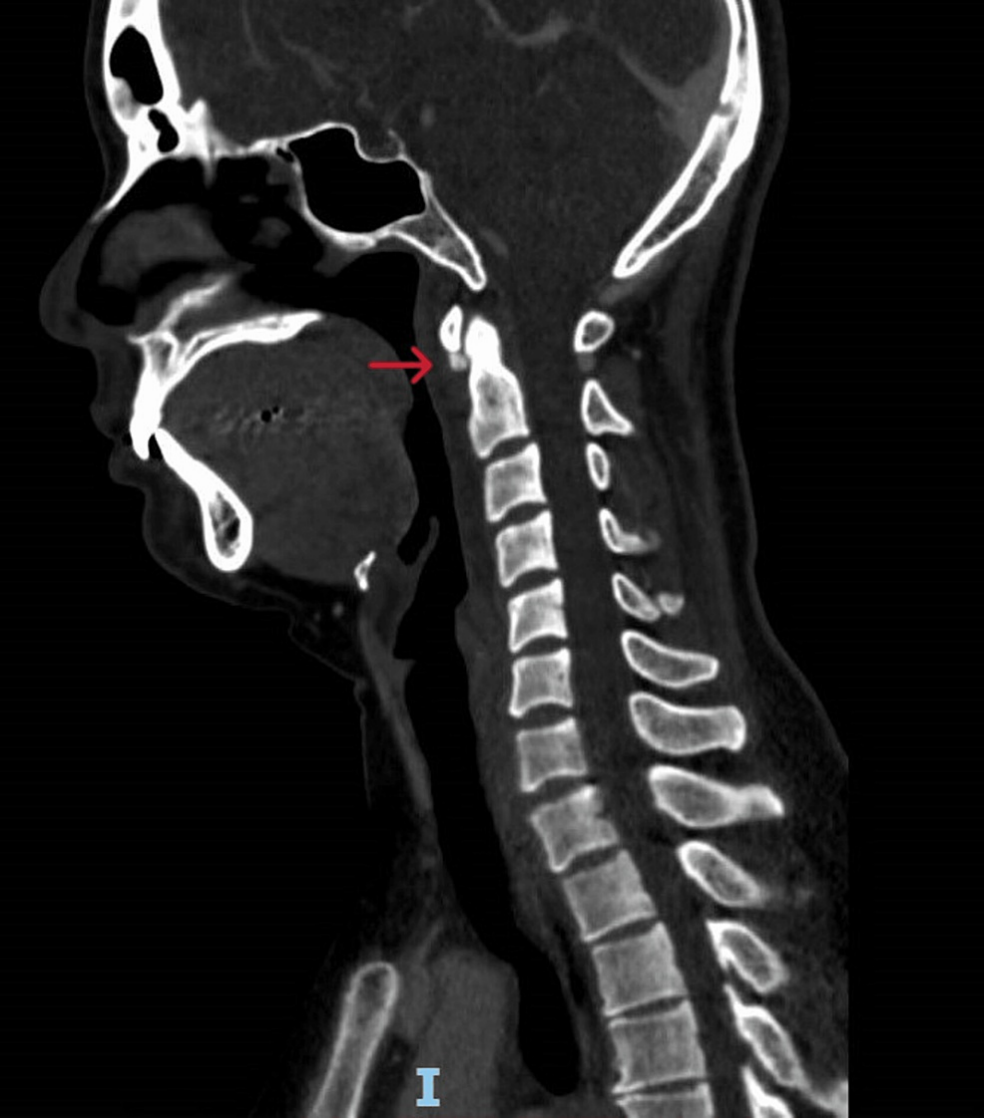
CT scan of the neck demonstrating an amorphic calcific density anterior to the C1 vertebra
